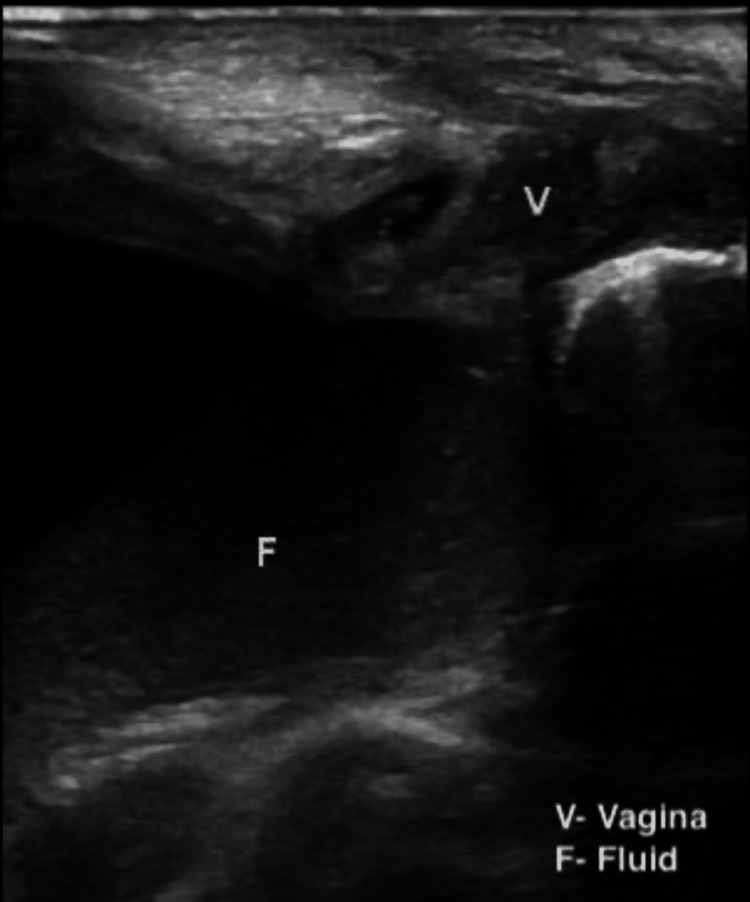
Ultrasound showing a pelviabdominal cyst composed of turbid fluid content arising from the vagina.
